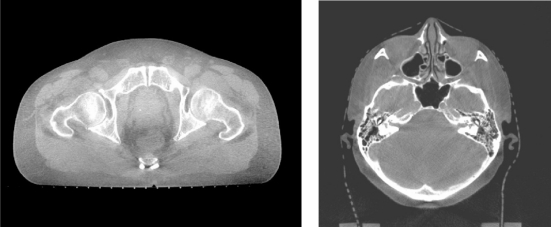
CT images produced by the cone beam CT system of a linear accelerator (courtesy of Professor Lei Xing, Stanford University School of Medicine, USA)
